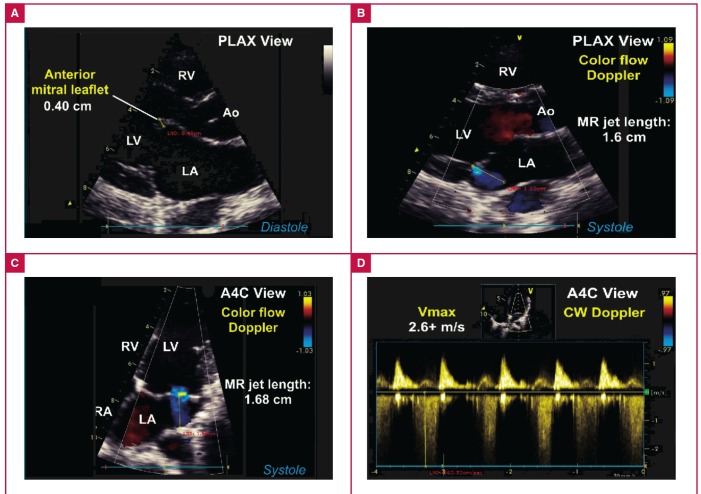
Example of borderline RHD consistent with WHF 2012 criteria. A. Parasternal long-axis (PLAX) view showing thickened anterior mitral leaflet exceeding 3 mm and restricted leaflet motion in young adult study subject. B. Colour-flow Doppler interrogation of the mitral valve in the PLAX view shows a small jet of mitral regurgitation (MR) with jet length < 2 cm. C. Colour-flow Doppler interrogation of the mitral valve using the A4C view shows jet of mitral regurgitation with jet length < 2 cm. D. Continuous-wave (CW) Doppler interrogation of the MR jet showed maximum velocities exceeding < 3 m/s.
